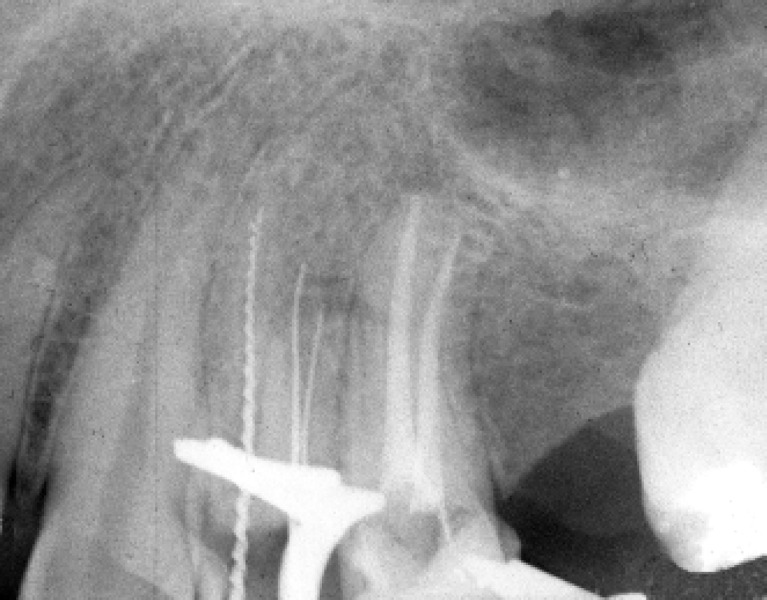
Initial radiograph, revealing two canals with a vague outline for the buccal root, three canals were negotiated
